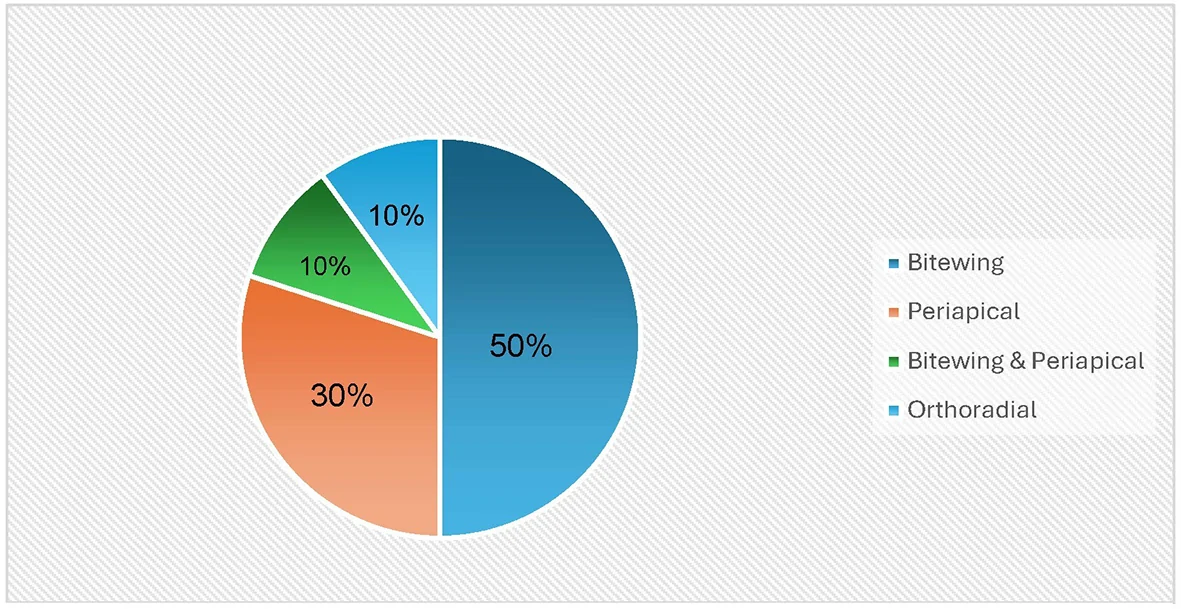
Distribution of radiograph types among included studies.
